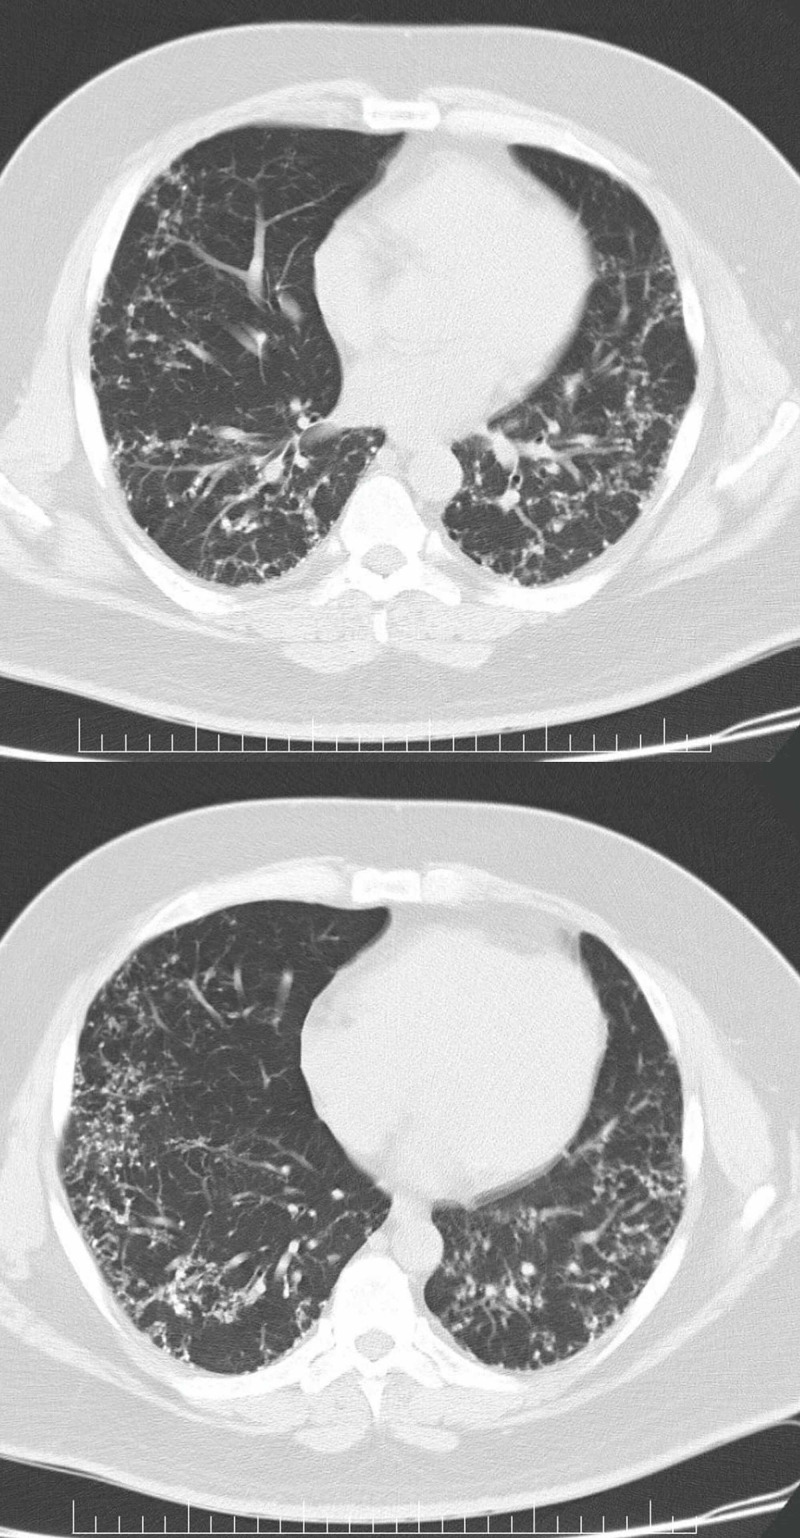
High-resolution computed tomography (CT) scan of the chest (at the heart level) The above CT images demonstrate nodular thickening of the interlobular septa more prominent in the sub-pleural regions in a lattice-like appearance. Also, dense nodules can be observed along the interlobular septa and in the sub-pleural interstitium.
